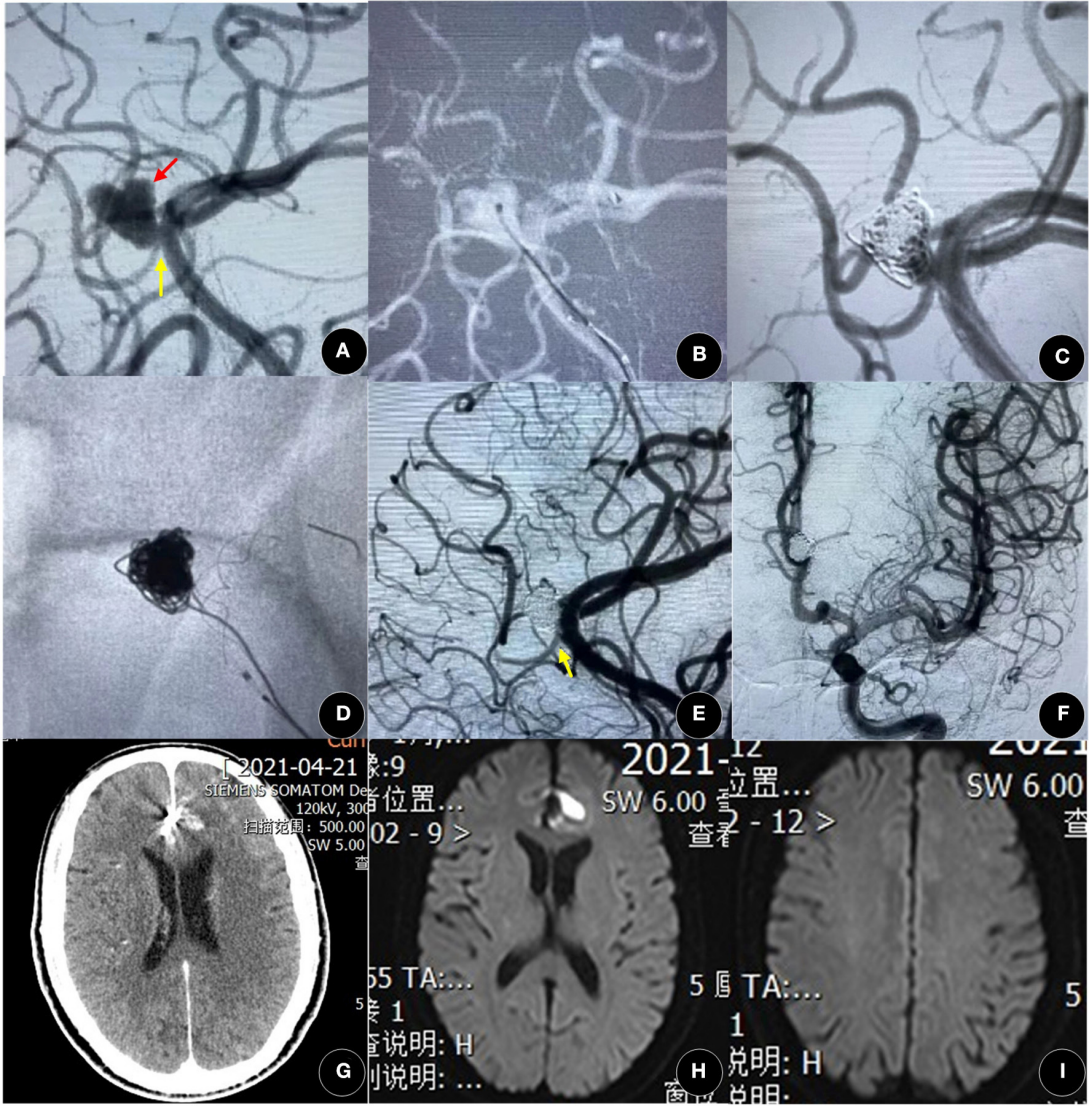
A 60-year-old woman presented with spontaneous SAH for 3 days. (A) The angiographic result showed a left lobular A3 aneurysm (black arrow) with a side branch (yellow arrow). (B,C) After coiling, the aneurysm was in incomplete occlusion [Raymond-Ray Occlusion Class (RROC) 3c]. (D–F) The aneurysm was achieved in complete occlusion (RROC 1) after the deployment of the Leo plus baby stent (2.5 mm × 18 mm), and the side branch was not affected (yellow arrow). (G–I) There was no new cerebral hemorrhage or infarction by computed tomography (CT) and magnetic resonance imaging (MRI). A3, the third segment of the anterior cerebral artery; SAH, subarachnoid hemorrhage.
